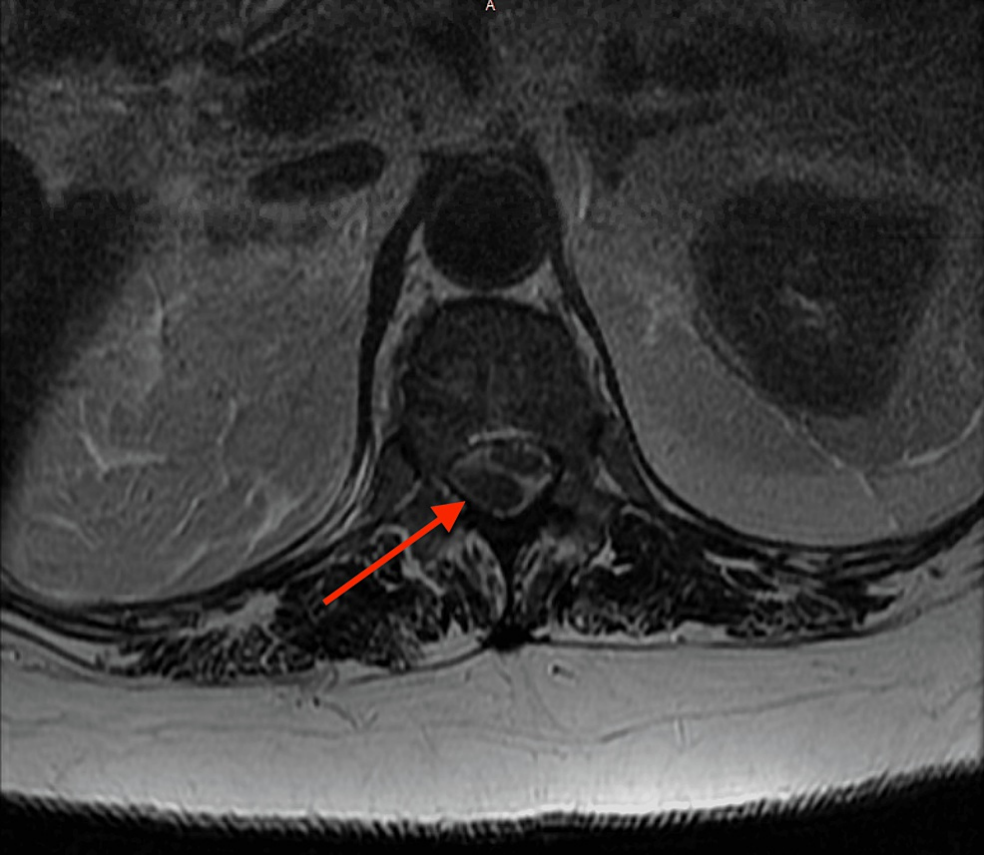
T2-weighted MRI of the thoracic spine with IV contrast; axial view
